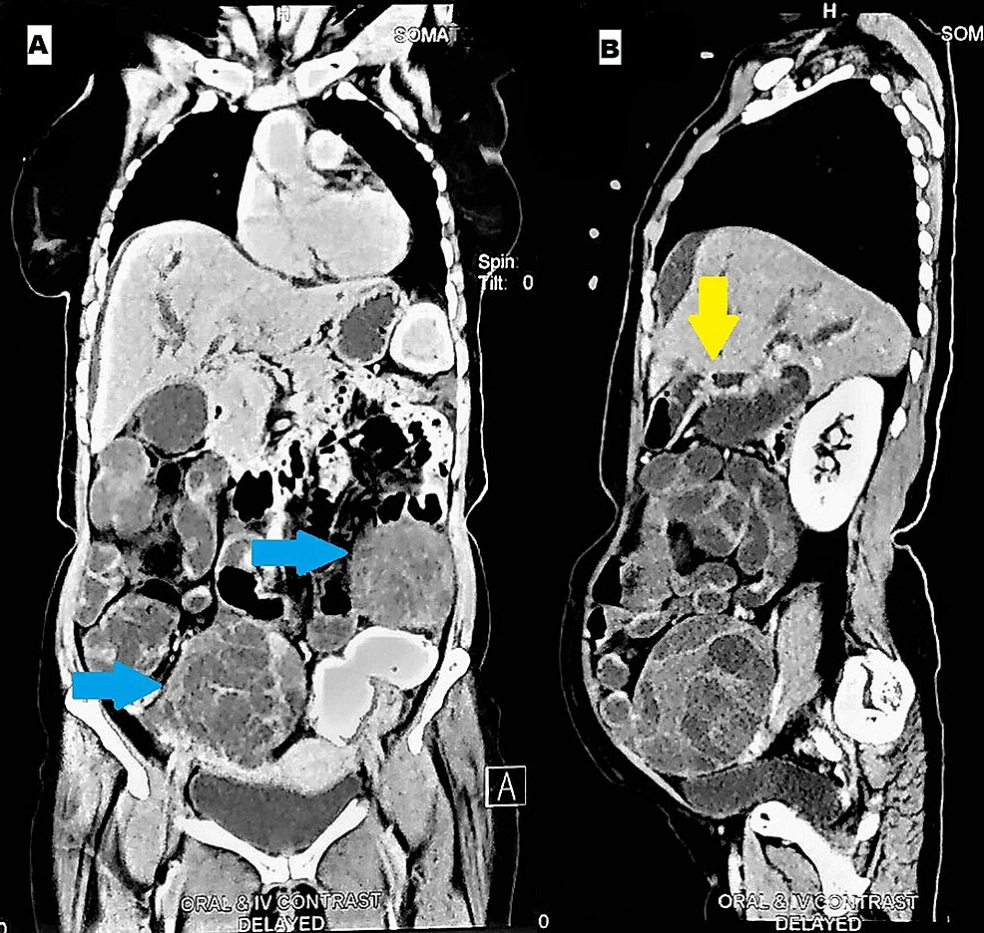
Abdominal computed tomography scan in delayed phase showing coexistent proximal bile duct cholangiocarcinoma (yellow arrow) and bilateral adnexal masses (blue arrows) in (A) coronal and (B) sagittal sections.
